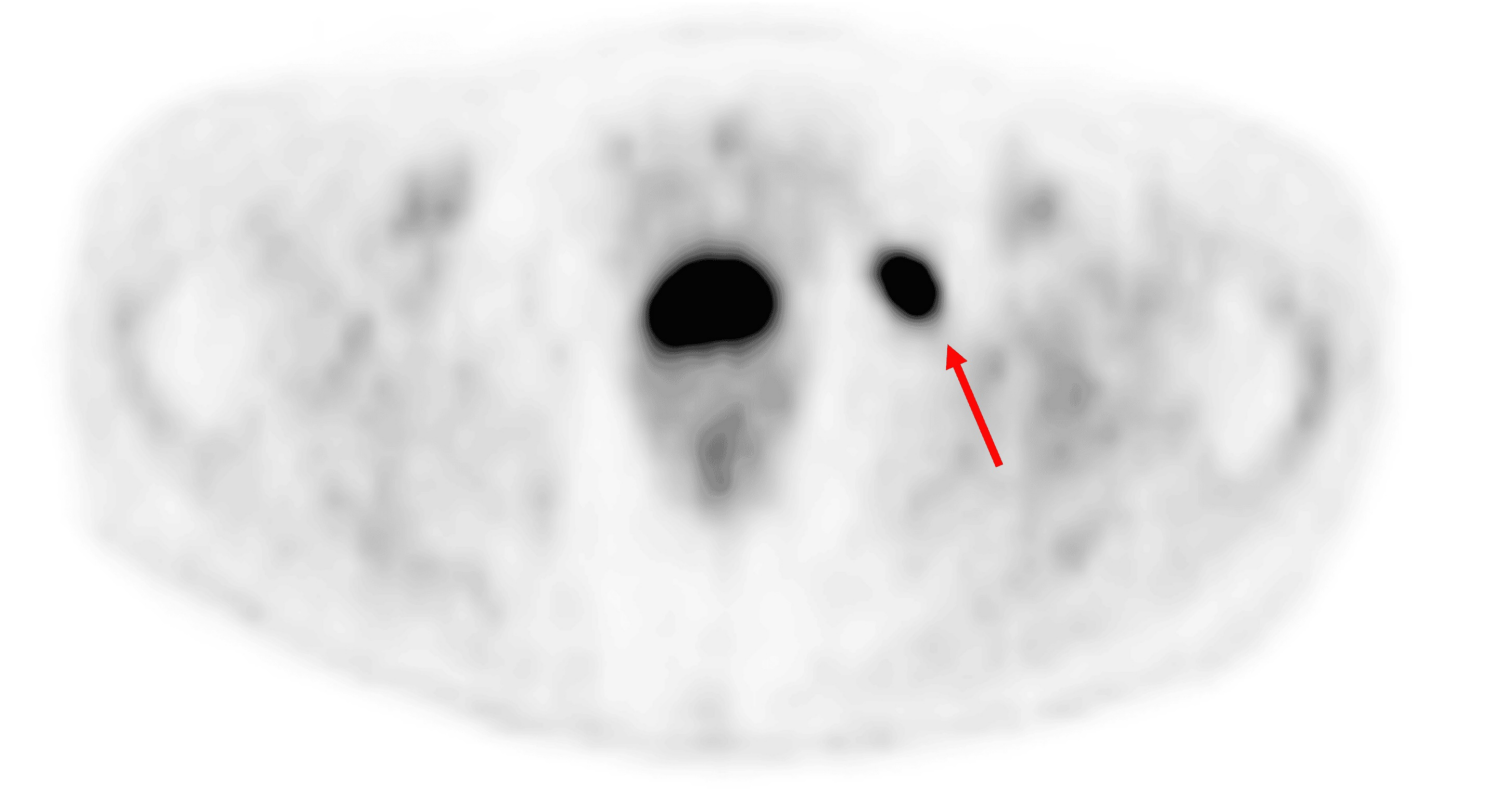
Positron emission tomography image demonstrating an fludeoxyglucose-avid area (maximum standardized uptake value of 11.6) of concern in the left pelvis. This was described as an avid “lymph node” on outside imaging and was the target requested for a computed tomography-guided biopsy.
